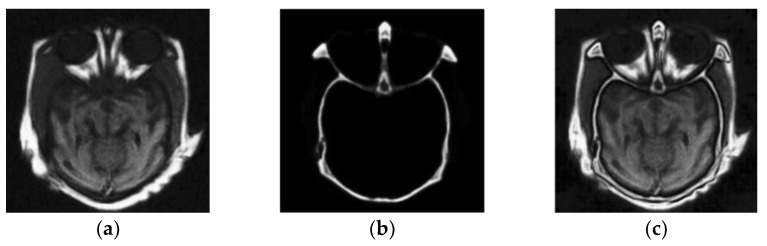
Image fusion in the field of clinical imaging: (a) MRI; (b) CT; (c) fusion image.
